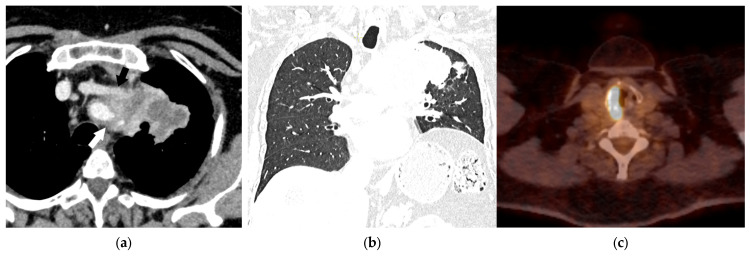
T4 category: Axial contrast-enhanced chest CT image showing lung cancer in the upper-left lobe invading the mediastinum, left brachiocephalic vein (black arrow), and supra-aortic arteries (white arrow, (a)). The elevated left hemidiaphragm confirms phrenic nerve invasion or at least the T3 category (b). There is increased FDG uptake in the right vocal cord as a result of the left recurrent laryngeal nerve invasion (T4 category), with an increased workload of the right-sided vocal cord muscles (c).
